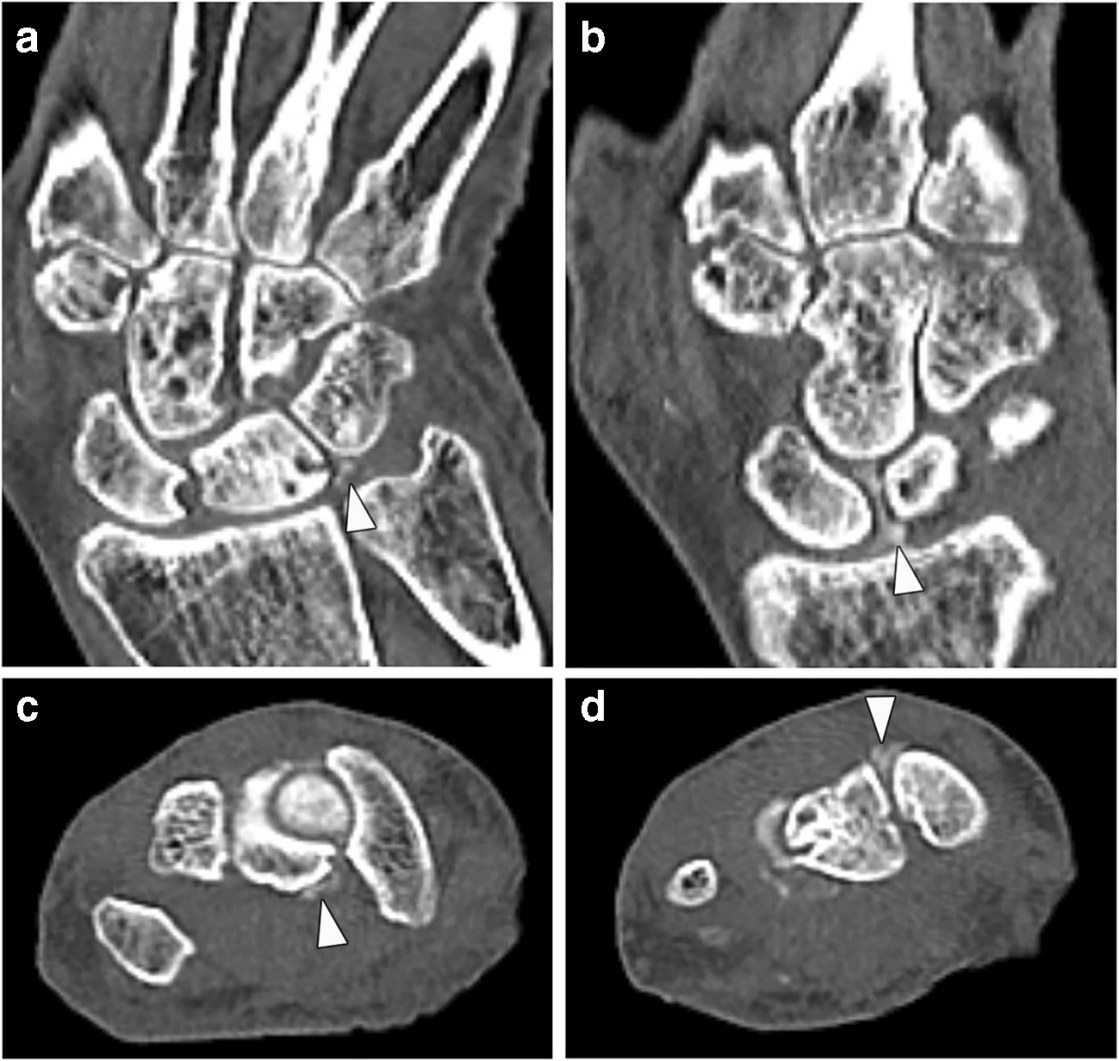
Imaging examples: ligamentous calcifications. Computed tomography of an 80-year-old female patient with CPPD; 80 keV bone kernel reconstructions. a Coronal reconstruction; arrowhead indicating calcification in proximal portion of the LT ligament. b Coronal reconstruction; arrowhead indicating calcification in proximal portion of the SL ligament. c Axial reconstruction; arrowhead indicating calcification in palmar portion of the SL ligament. d Axial reconstruction; arrowhead indicating calcification in dorsal portion of the SL ligament
